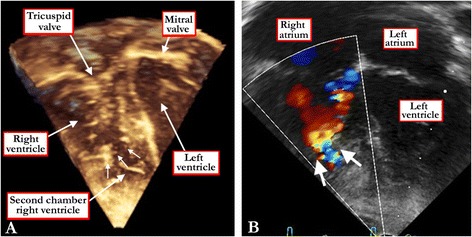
The three dimensional echocardiographic dataset, shown in four chamber fashion (A), reveals abnormal muscle bundles in the right ventricle (white arrows), producing so-called double chambered right ventricle. The Doppler color flow map in the same heart (B) confirms the presence of the double chambered right ventricle.
